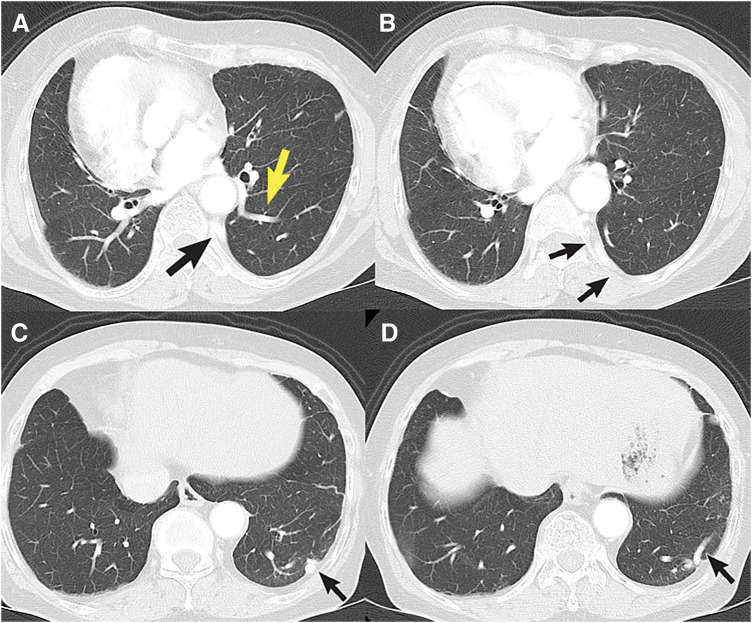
Chest CT (lung window). (A) Origin of the aberrant artery arising from the descending aorta (black arrow) and a dilated pulmonary vein branch (V10, yellow arrow). (B) Course of the aberrant artery running along the chest wall posterior to the parietal pleura (black arrows). (C) Entry point of the aberrant artery into the lung parenchyma (black arrow). (D) Intrinsic branches of the aberrant artery within the lung parenchyma (black arrow).
